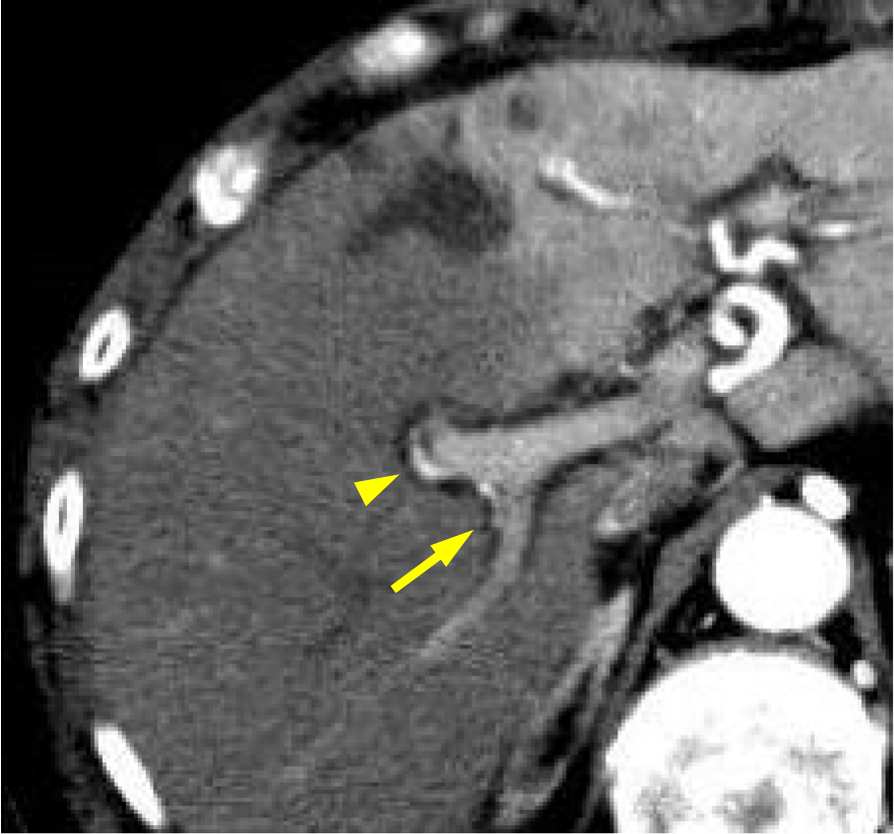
Postoperative imaging studies using contrast-enhanced CT at (A) postoperative day 1 and (B) 5 months after operation. (A) Arrowhead and arrow indicate anterior and posterior sectorial branch of the right hepatic artery, respectively.
